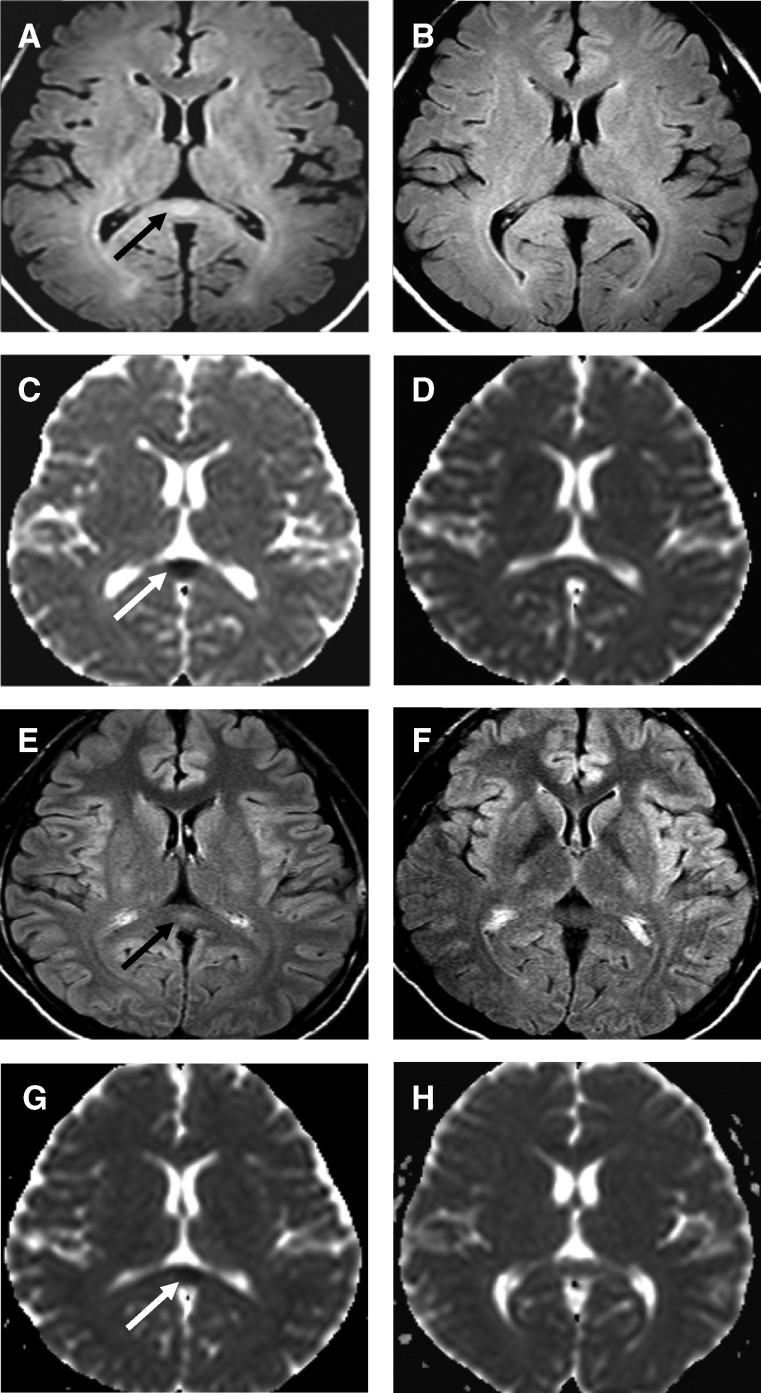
Magnetic resonance imaging studies of two patients with acute malaria who had no neurologic symptoms or signs. A–D, A 30 year-old man with uncomplicated malaria and a mixed infection (*Plasmodium falciparum*: 9,200 parasites/μL; *P. vivax*: 128 parasites/μL). E–H, A 22 year-old man with hyperparasitemia (*P. falciparum*: 588,000 parasites/μL). T2-weighted fluid-attenuated inversion recovery sequences (A and E) obtained shortly after admission show hyperintense, symmetrical oval lesions in the midline of the splenium of the corpus callosum (black arrows). Repeat examinations four weeks later (B and F), show resolution of the lesions. Diffusion-weighted imaging echo-planar imaging studies (C and G) shortly after admission showed relative decreases in the apparent diffusion coefficient within the lesions (white arrows) that had also resolved on the repeat studies (D and H) four weeks later.
